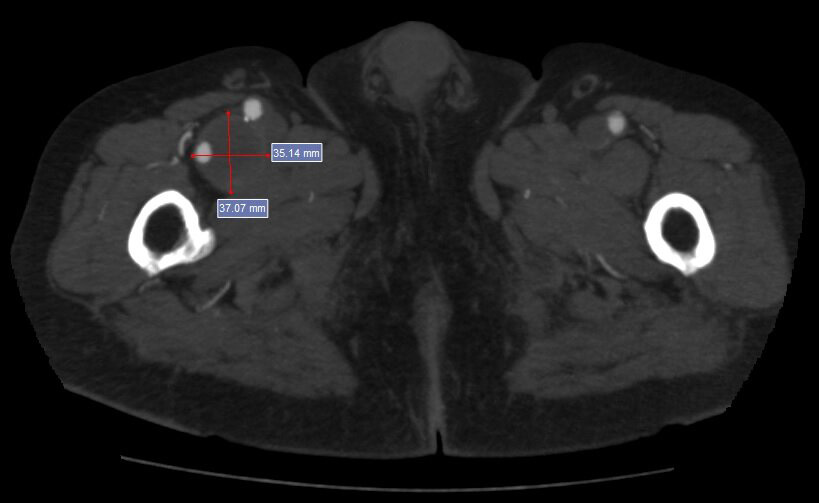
Axial image from computed tomography angiography (CTA) of the proximal thigh, showing an aneurysmal dilatation of the deep femoral artery (DFA) with diameters of 3.7 cm (anteroposterior) and 3.5 cm (lateral) and intraluminal thrombi.
